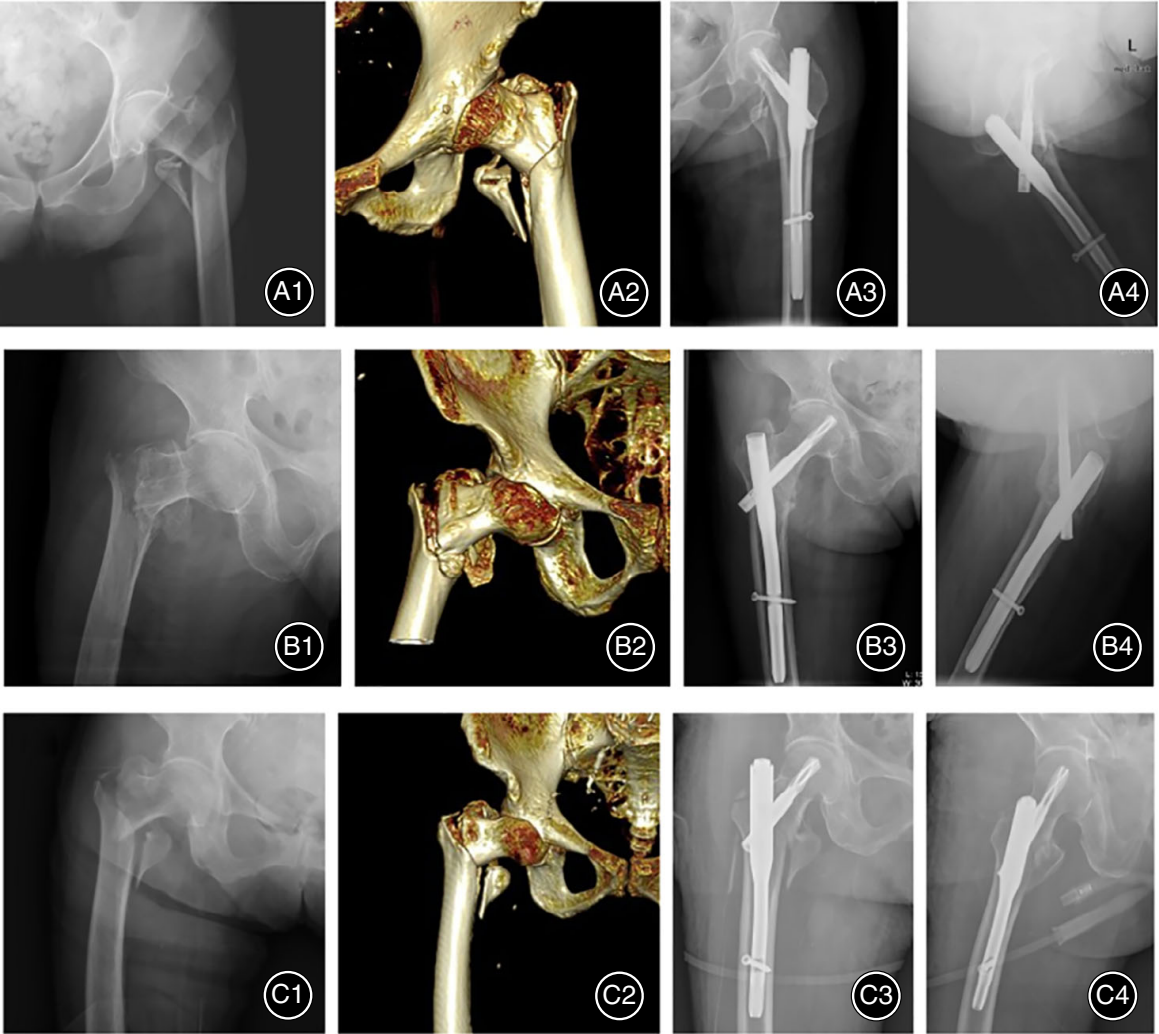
Preoperative X‐rays (A1, B1, C1) and CT scans (A2, B2, C2) of femoral intertrochanteric fracture from three patients ((A) 77‐year‐old female; (B) 84‐year‐old female; (C) 78‐year‐old male), and which had been treated by closed reduction and internal fixation with PFNA (A3, A4, B3, B4, C3, C4).
